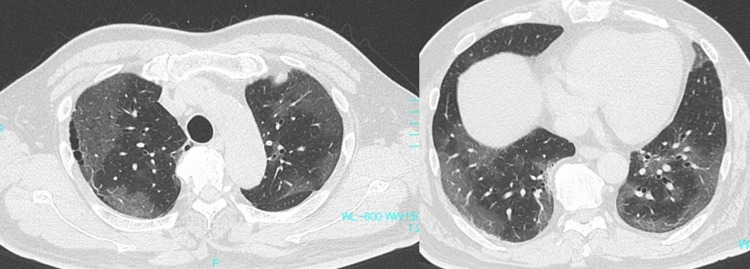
Chest CT findings on admission Bilateral ground glass opacity in peripheral lungs were seen upon admission.
